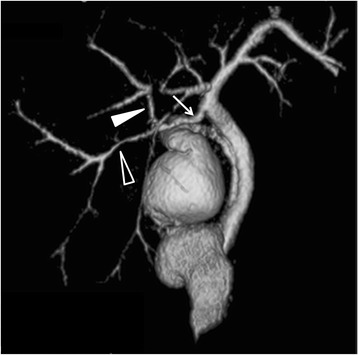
Three-dimensional DIC-CT (right ventral view) showing a type I accessory hepatic duct. The accessory hepatic duct (*arrow*) drained both the dorsal area of the right anterior section (*white arrow head*) and the right posterior section (*black arrow head*) and was classified into the combined posterior and anterior branch type
